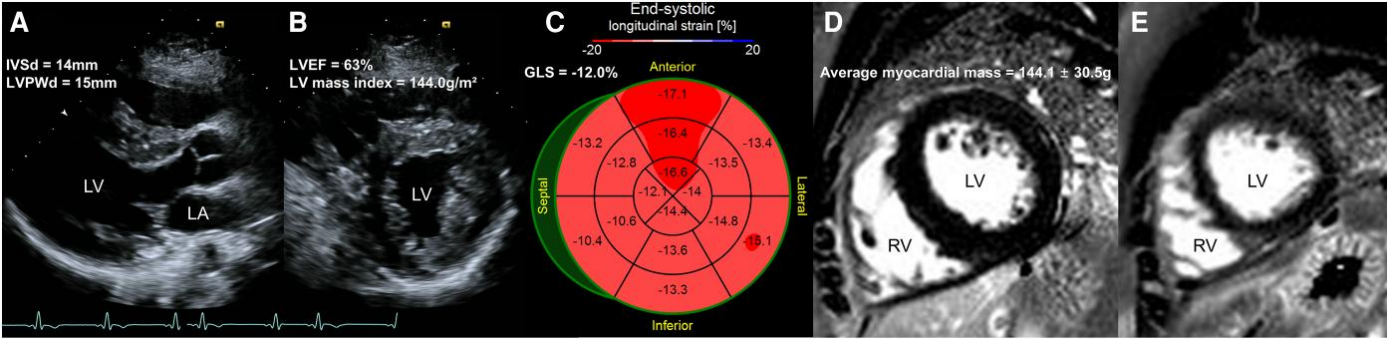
(*A*, *B*) Transthoracic echocardiography demonstrates left ventricular hypertrophy, with interventricular septal thickening (14 mm) and increased posterior wall thickness (15 mm), consistent with concentric hypertrophy (*C*) global longitudinal strain: bull's eye plot. (*D*, *E*) Cardiac magnetic resonance image reveals normal myocardial thickening and left ventricle mass with no late gadolinium enhancement. GLS, global longitudinal strain; IVSd, interventricular septal diameter; LA, left atrium; LV, left ventricle; LVEF, left ventricular ejection fraction; LVPWd, left ventricular posterior wall diameter; RV, right ventricle.
